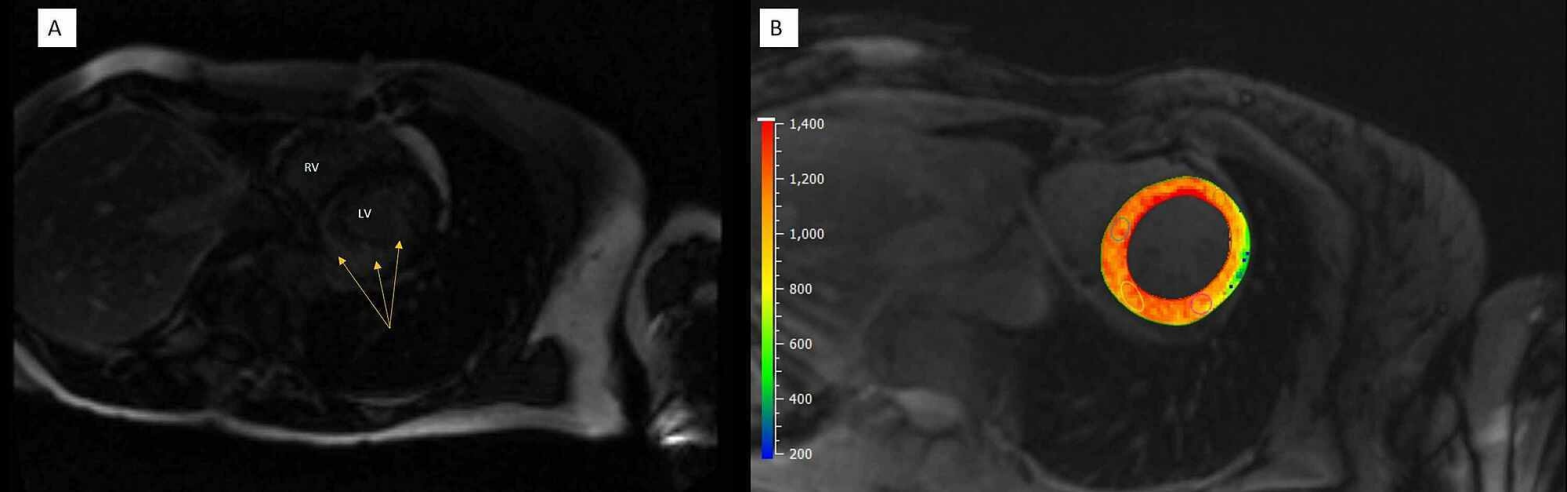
Cardiac MRI. A: Short axis view after gadolinium contrast administration. There is patchy delayed enhancement involving the mid-myocardial inferior wall. RV = right ventricle; LV = left ventricle. B: Short axis view T1 map showing significantly elevated T1 values highly suggestive of cardiac amyloid. Normal myocardium T1 value is 900 ms-1,000 ms on a 1.5T scanner.
